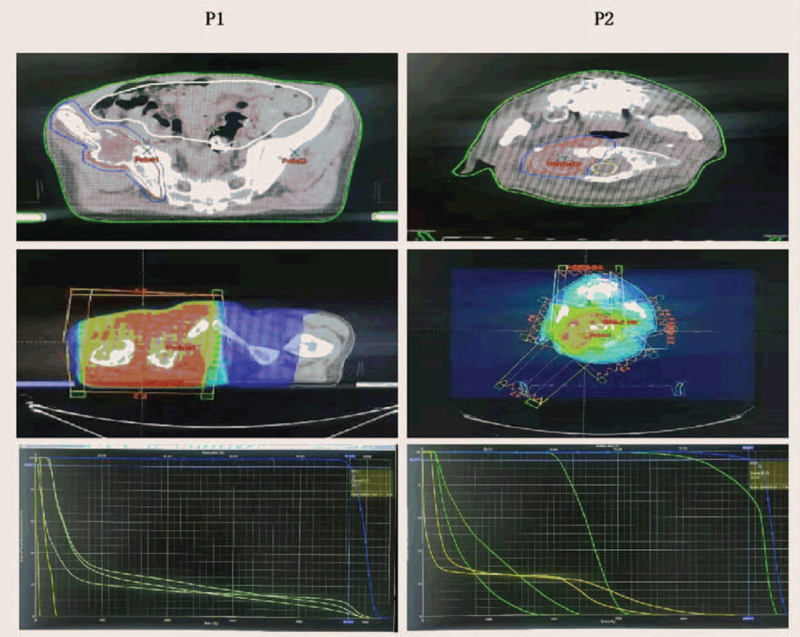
External radiotherapy for bone metastases from April 2019 to May 2019. The specific radiotherapy regimen was as follows: 6 mV X-ray, planning target volume covered by the 95% isodose curve, DT 5000 cGy/25 f/5 w (P1, right iliac bone), and DT 5000 cGy/25 f/5 w (P2, skull base and first cervical vertebra).
